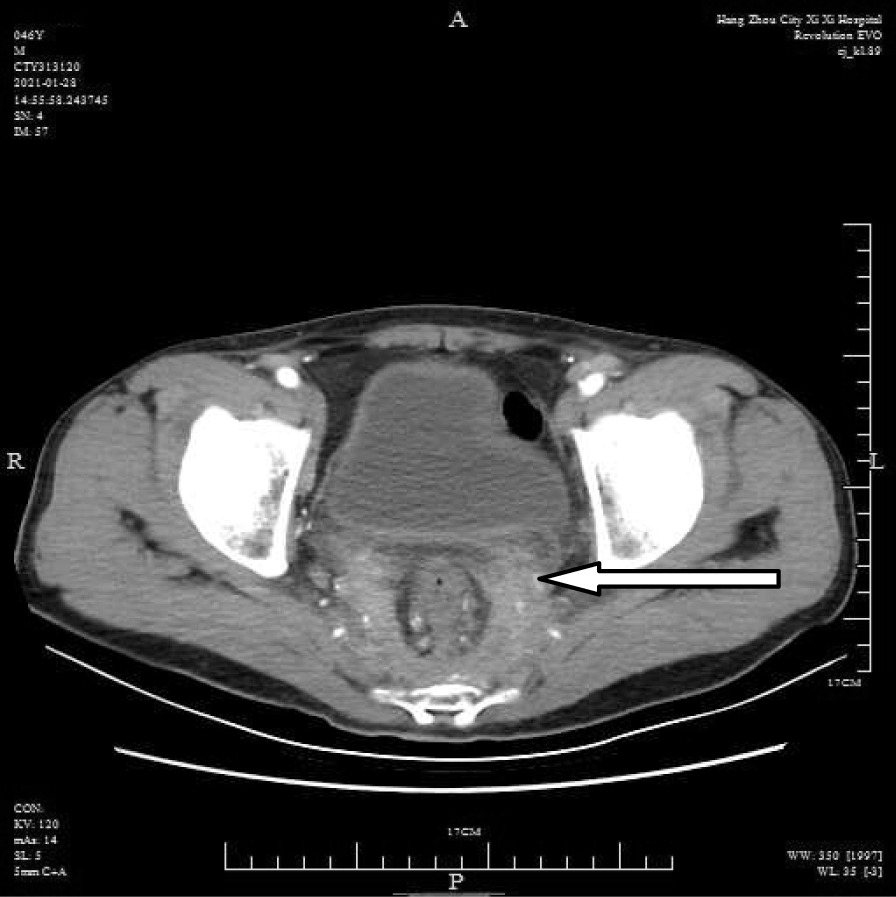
Retroperitoneal fibrosis in CT before the treatment
